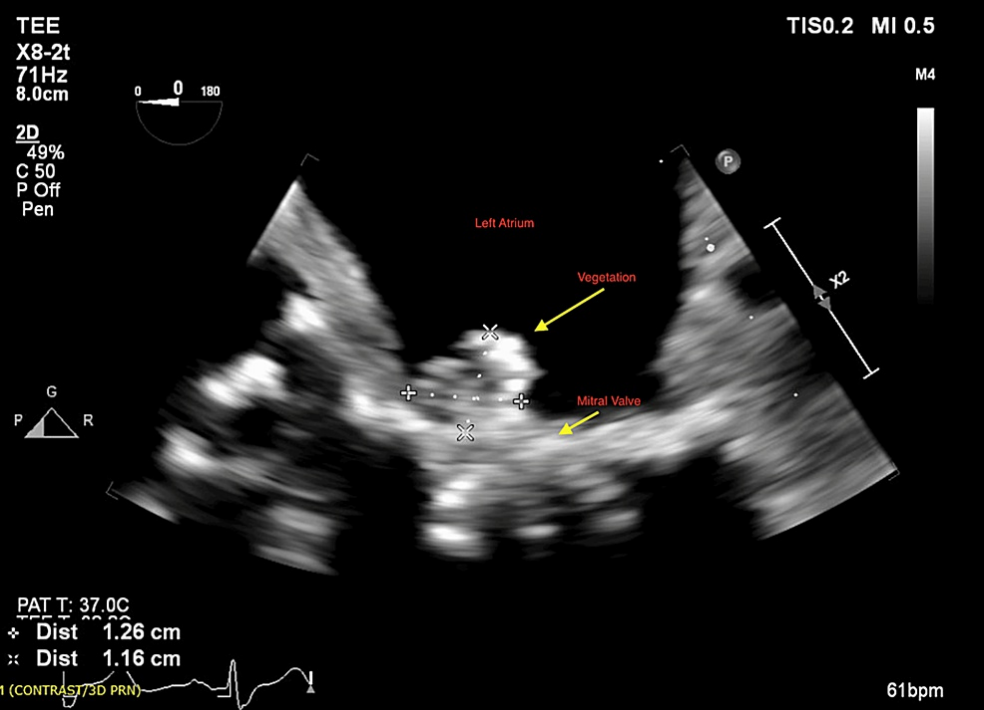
Transesophageal echocardiogram showing a large mobile vegetation (1.3 cm) on the anterior leaflet of the mitral valve
